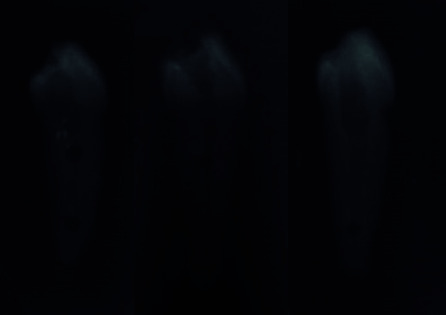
Radiografía periapical de las perforaciones simuladas a diferentes
